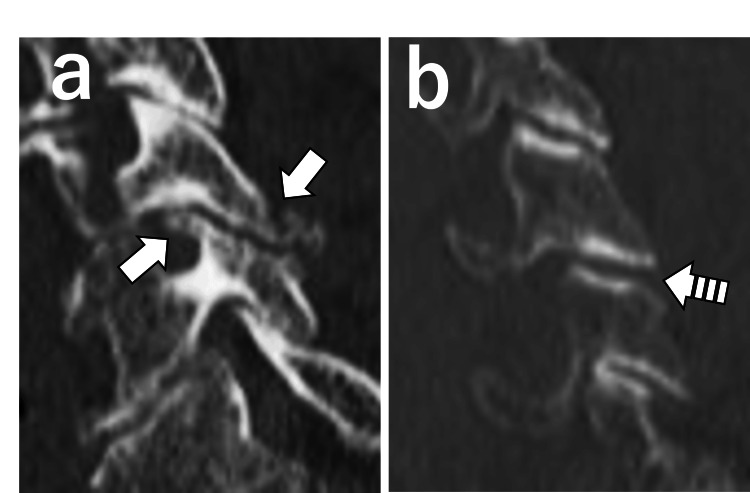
Parasagittal CT images. (a) Right-sided parasagittal image showing fractures of the superior and inferior articular processes (arrows). (b) Contralateral parasagittal image showing facet joint widening (striped arrow).
